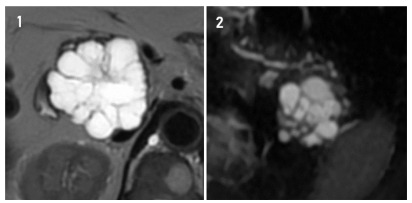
Two patients with typical SCP, both on MRI. 1: A microcystic lesion
